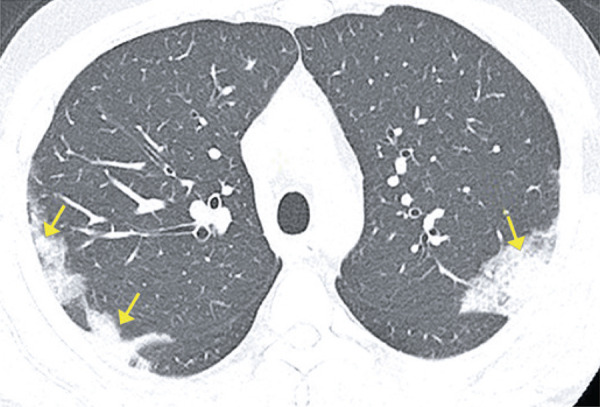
A 41-year-old patient with COVID-19, presenting cough, fever and dyspnea for five days. Computed tomography showing peripheral consolidations in the upper lobes
